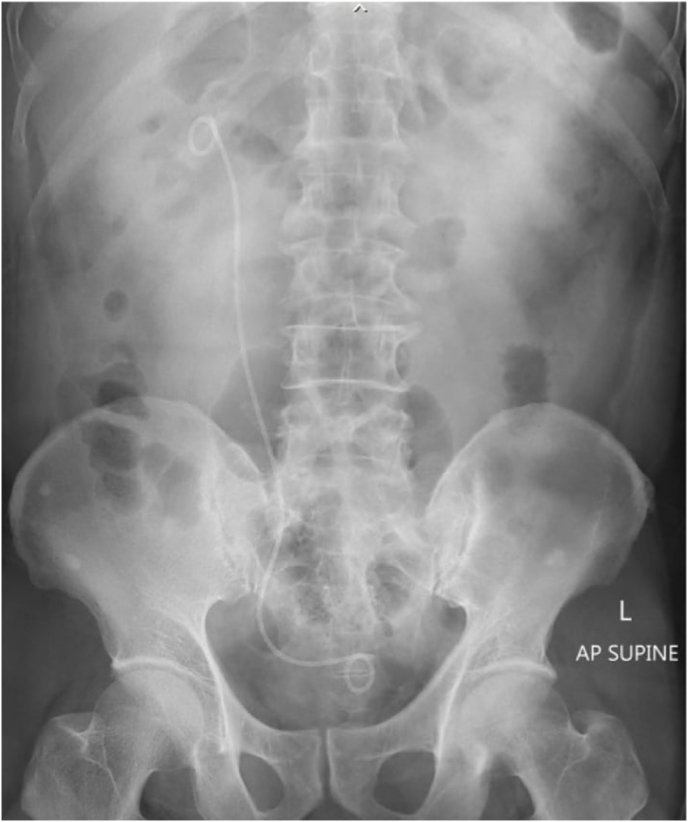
Post-operative abdominal plain radiography showing no evidence of residual calculi.
